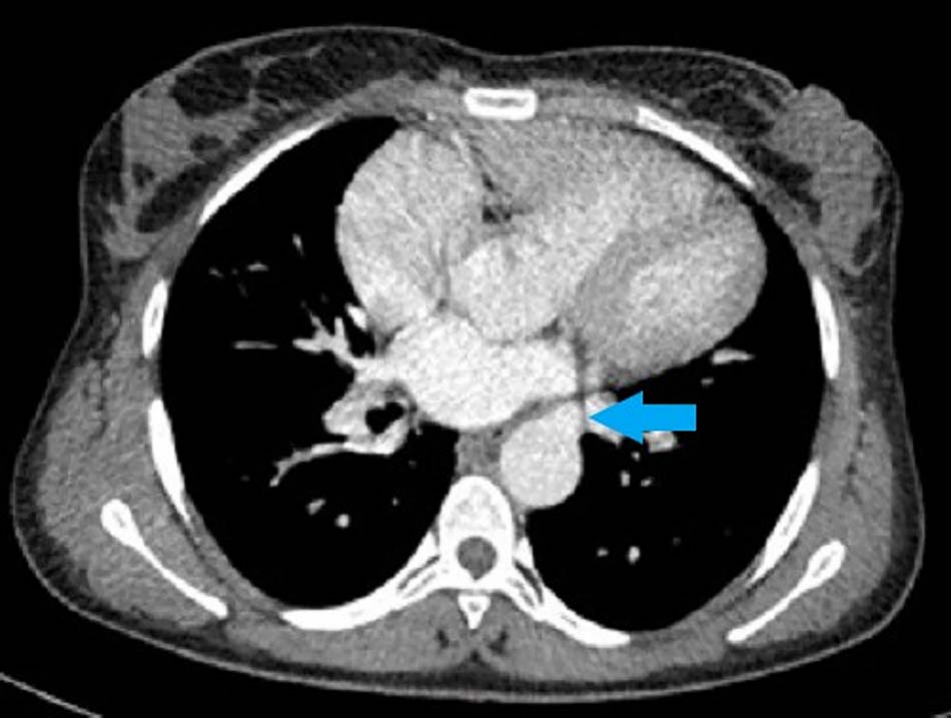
CT chest axial view shows third collateral artery (blue arrow) arising from the left margin of the descending aorta at the level of the left atrium.
